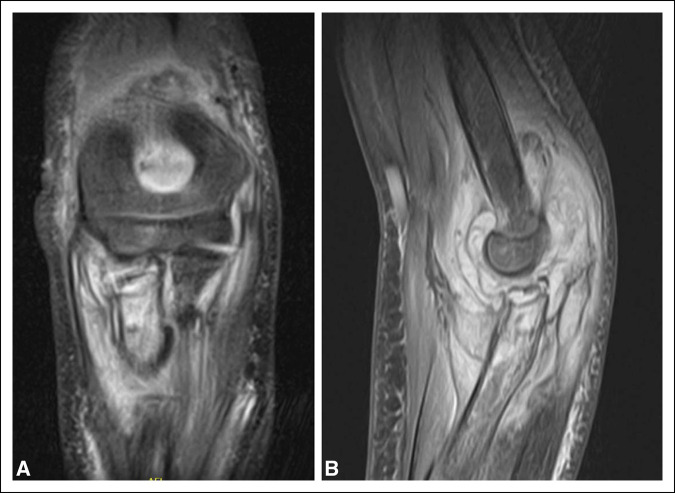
Radiographs showing T2 coronal and sagittal MRI slices concerning for elbow septic arthritis and osteomyelitis in the proximal ulna.
